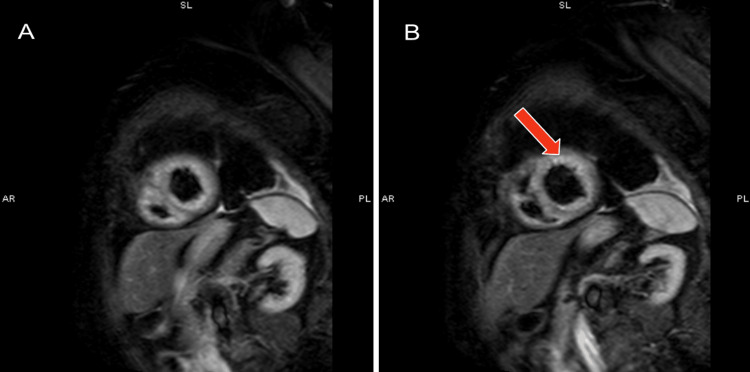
(A and B) T2-weighted MR images of the apical segments of the left ventricle showing evidence of inflammation demonstrated by bright white portions of the myocardium (abnormal) compared to the dark gray portions of the myocardium (normal).
